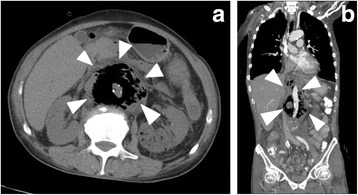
a Axial view, and b coronal view contrast-enhanced computed tomography scan images showing an aneurysm with periaortic gas (the area immediately outside the gas-filled region is the tunica adventitia of the aorta; shown by *arrowheads*)
